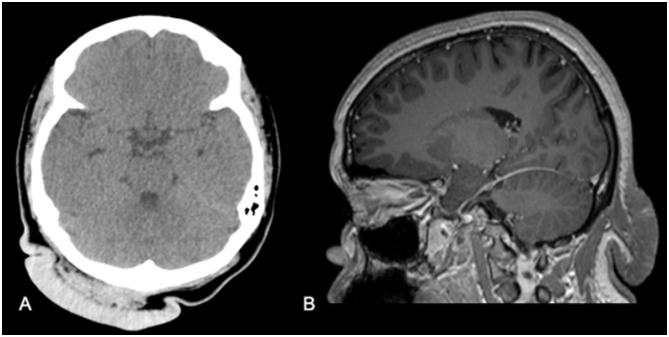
CT (A) and MRI scans (B) showing a soft tissue lesion characterized by marked cutaneous thickening in the occipital region.
